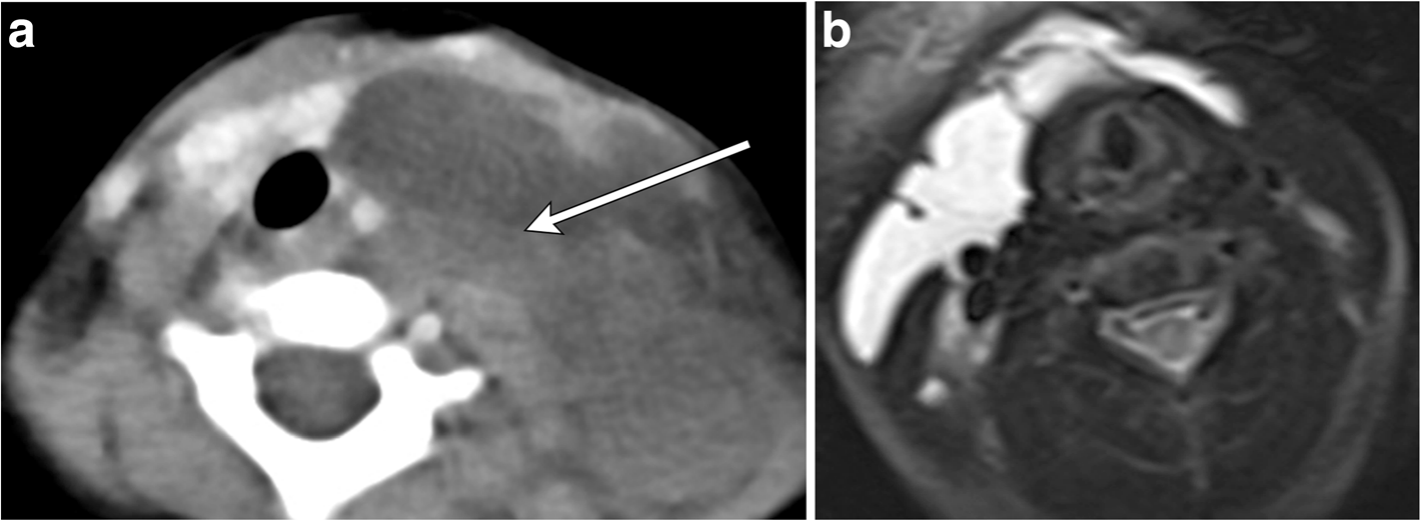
Lymphangioma. a Axial contrast-enhanced CT image demonstrates a non-enhancing multilocular, trans-spatial fluid-attenuation mass centered in the left neck base with mass effect and rightward displacement of trachea and esophagus. Note the fluid-fluid levels indicative of prior hemorrhage (arrow), distinguishing this lesion from a thyroglossal duct cyst. b Axial T2-weighted MR image in a different patient demonstrates a trans-spatial multiseptated cystic mass extending from the right lateral infrahyoid neck across the anterior aspect of the thyroid cartilage
